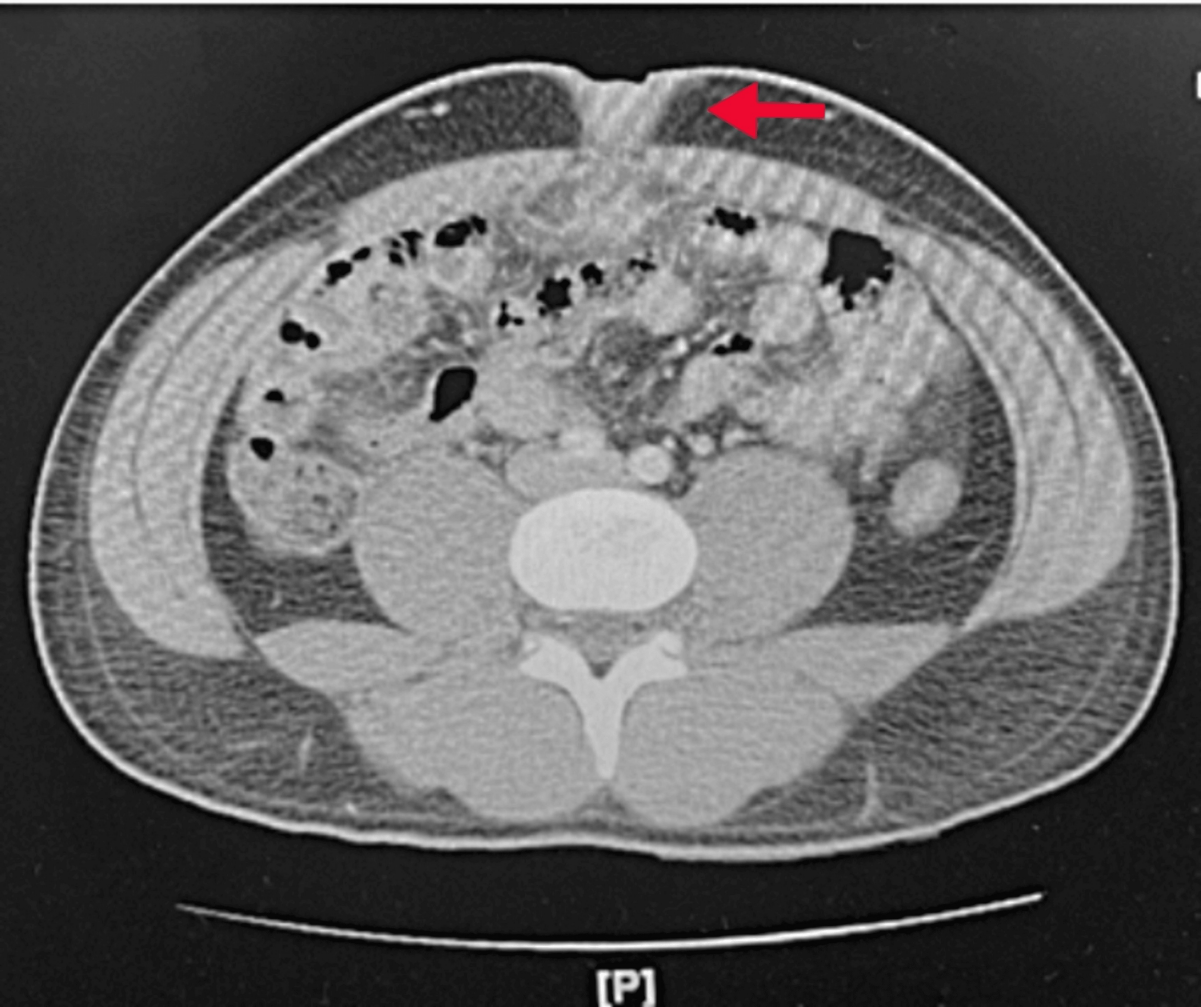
Transverse section CT abdomen pelvis
